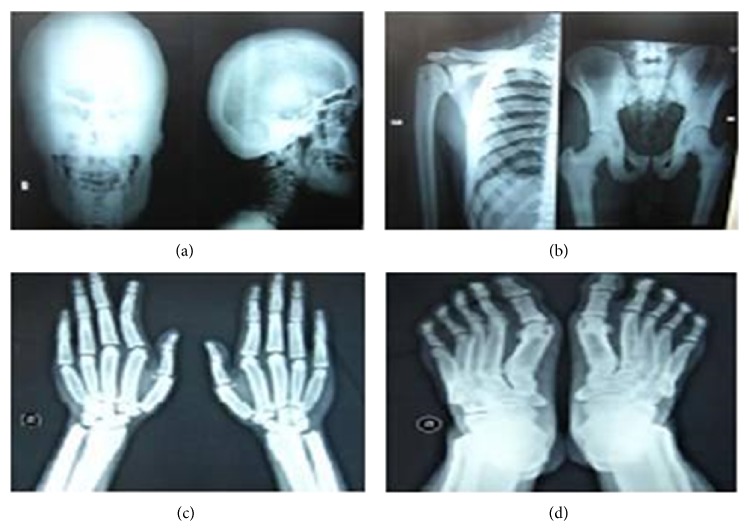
Radiological manifestations in patient 1. Skull X-ray (AP and lateral views) showing diffusely thickened dense calvarial bones of skull base and prognathism (a); X-ray chest (AP view) showing right clavicle and humerus and X-ray pelvis and upper part of both femora (AP view) with diffuse sclerotic texture and widened clavicles and ribs (b); X-ray of both hands (AP view) showing sclerotic appearance with medullary expansion of metacarpals and phalanges and hypoplastic terminal phalanges (c); X-ray of both feet (AP view) showing sclerotic appearance with medullary expansion of metatarsals and phalanges and hypoplastic terminal phalanges, bilateral hallux valgus, and overcrowded tarsal bones (d).
